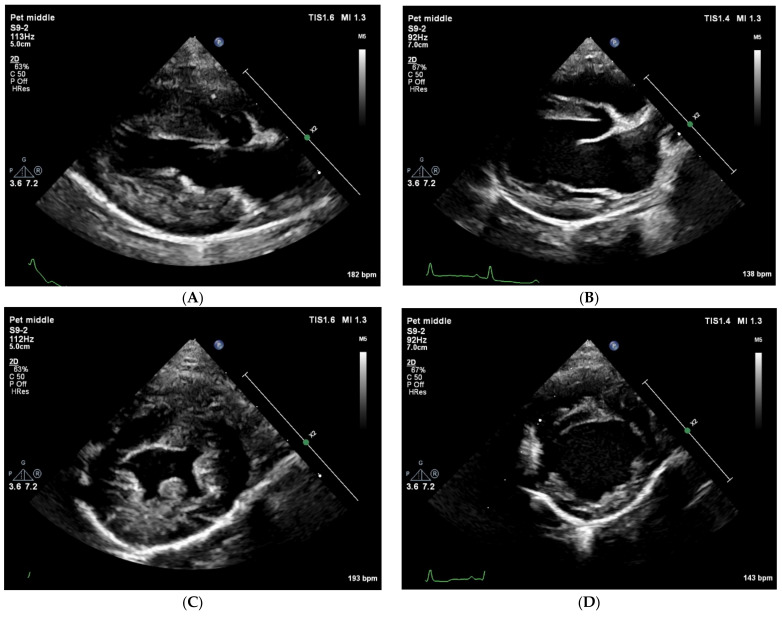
Transthoracic right parasternal two-dimensional grey-scale echocardiographic images at initial presentation (A,C) and 12 days later (B,D). All images show an end diastolic frame. Standard longitudinal four-chamber images (A,B) and standard short-axis images at the level of the papillary muscles (C,D) show at the initial presentation a severe underfilling of the left ventricle with severe concentric pseudohypertrophy, and normal wall- and lumen-dimensions 12 days later.
